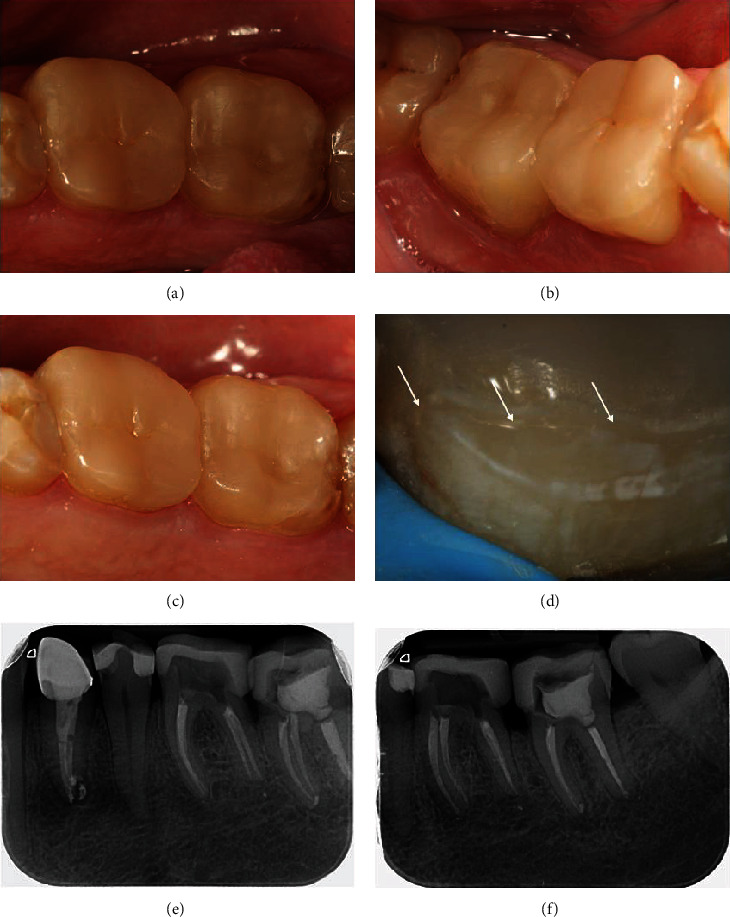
Four-year follow-up of the restorations: occlusal view (a); buccal view (b); lingual view (c); a close-up image of the overlay-tooth interface (d), white arrows pointing to the borders of the restorations; radiograph examination (e, f).
